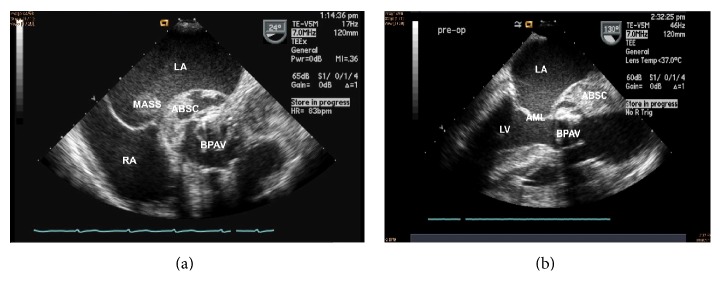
Transesophageal echocardiogram in short axis (a) and long axis (b) views, showing an extensive aortic root abscess around a bioprosthetic aortic valve, forming a fistulous communication to left atrium (legend: ABSC = abscess, LA = left atrium, MASS = mass in LA, BPAV = bioprosthetic aortic valve, RA = right atrium, AML = anterior mitral leaflet, and LV = left ventricle).
